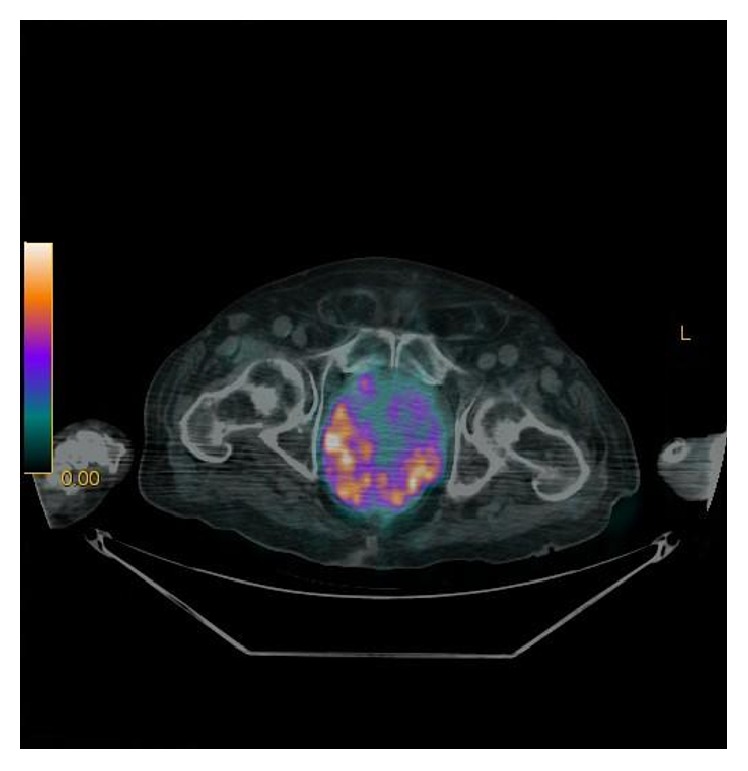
68 Gallium DOTATATE PET/CT image showing a high and diffuse uptake of the prostate.
